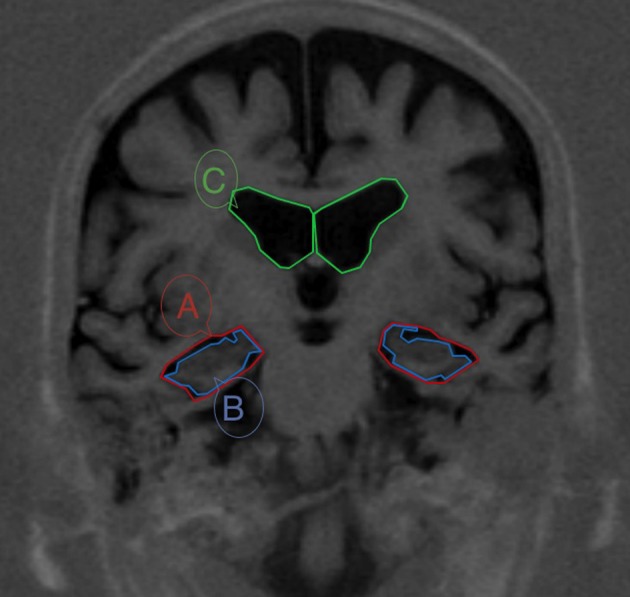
MRI from a patient with MCI with boundaries of the three areas needed for calculating the Medial Temporal Atrophy index (MTAi). The section passes through the interpeduncular fosae. The three areas are: (1) the medial temporal lobe region (A), defined in a coronal brain slide as the space bordered in its inferior side by the tentorium cerebelli, in its medial side by the cerebral peduncles, in its upper side by the roof of the temporal horn of the lateral ventricle and in its lateral side by the colateral sulcus and a straight-line linking the colateral sulcus with the lateral edge of the temporal horn of the lateral ventricle; (2) the parenchima within the medial temporal region, that includes the hippocampus and the parahippocampal girus (B); and (3) the body of the ipsilateral lateral ventricle (C).
